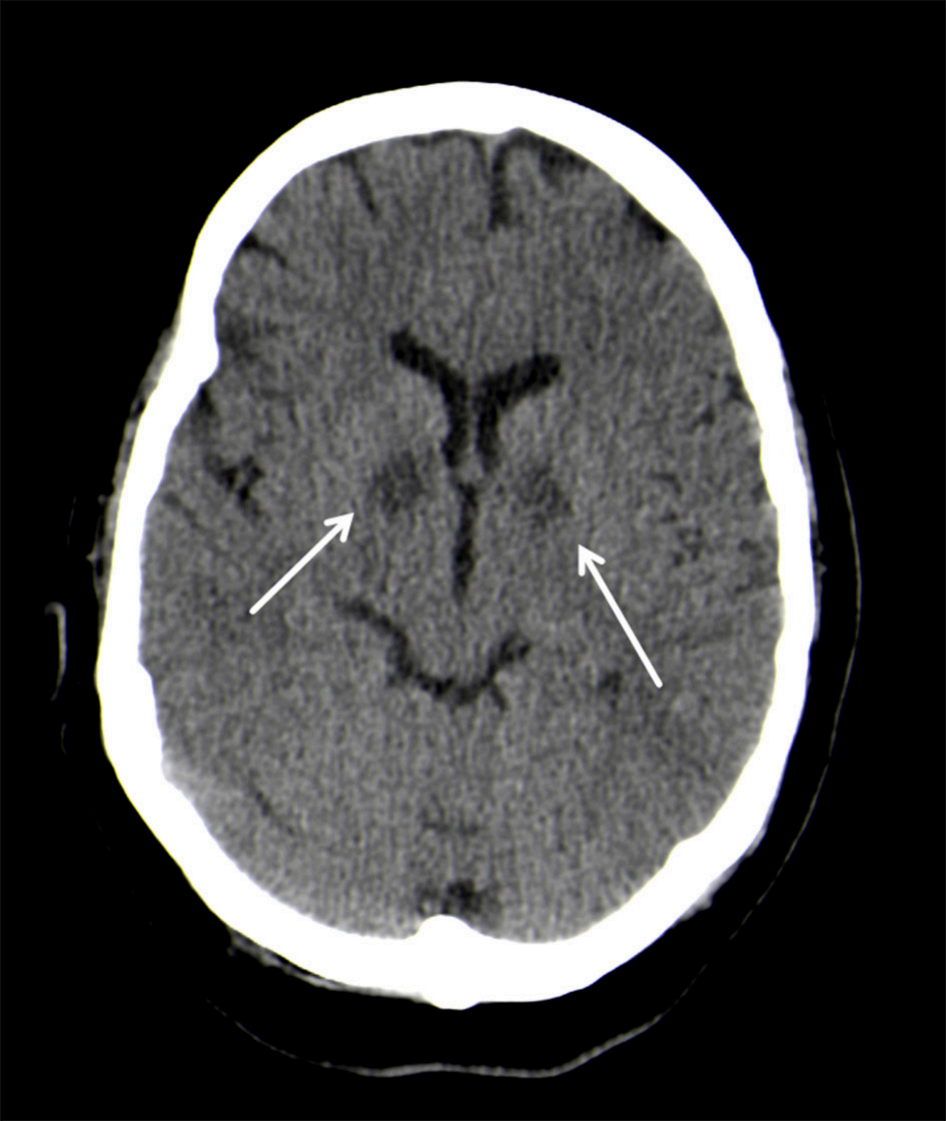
Bilateral, hypodense basal ganglia necrosis in unenhanced CT (arrows); Philips Ingenuity 5 mm.
